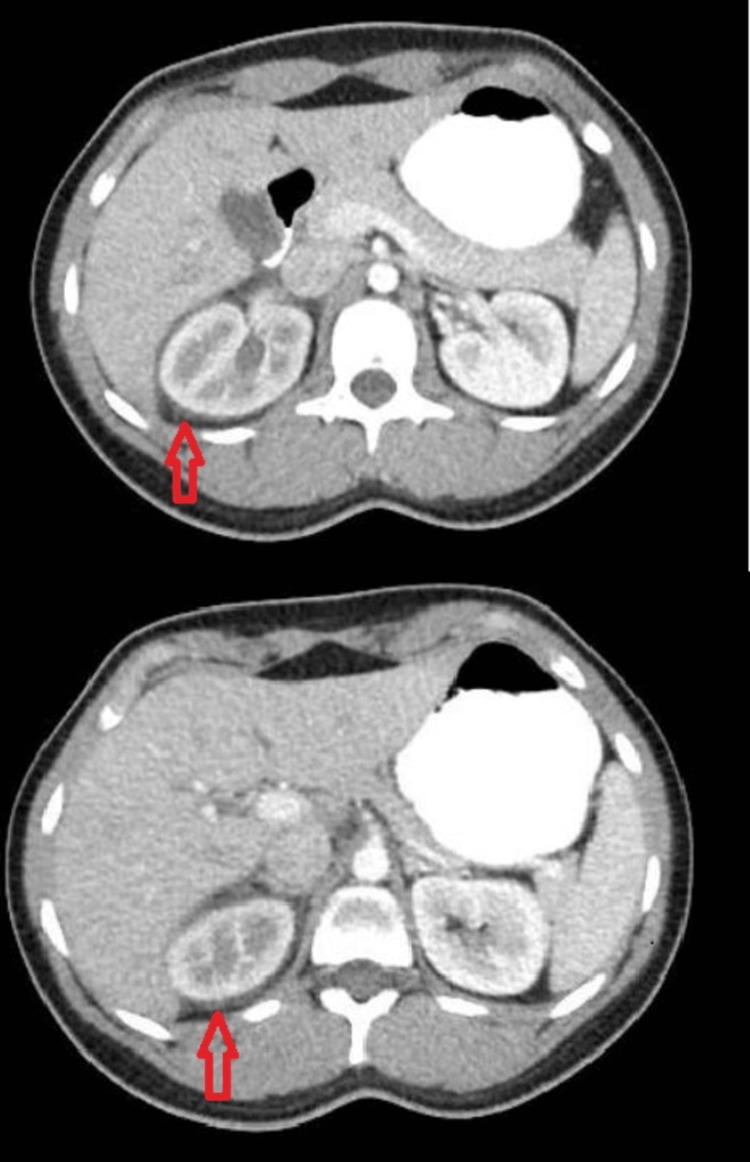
CT abdomen and pelvis with contrast shows perinephric edema/ fluid collection (arrowhead) around the right kidney
